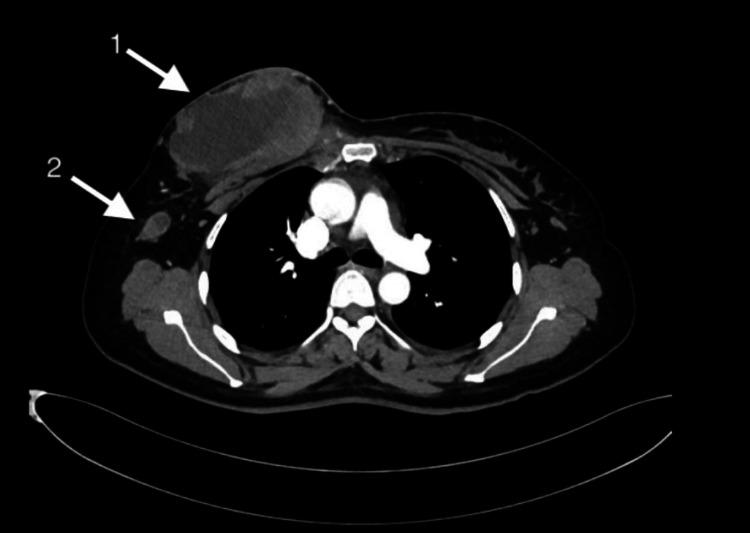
Computed tomography showing a large mass occupying all quadrants of the right breast (arrow 1) with large necrotic axillary lymphadenopathy (arrow 2)
